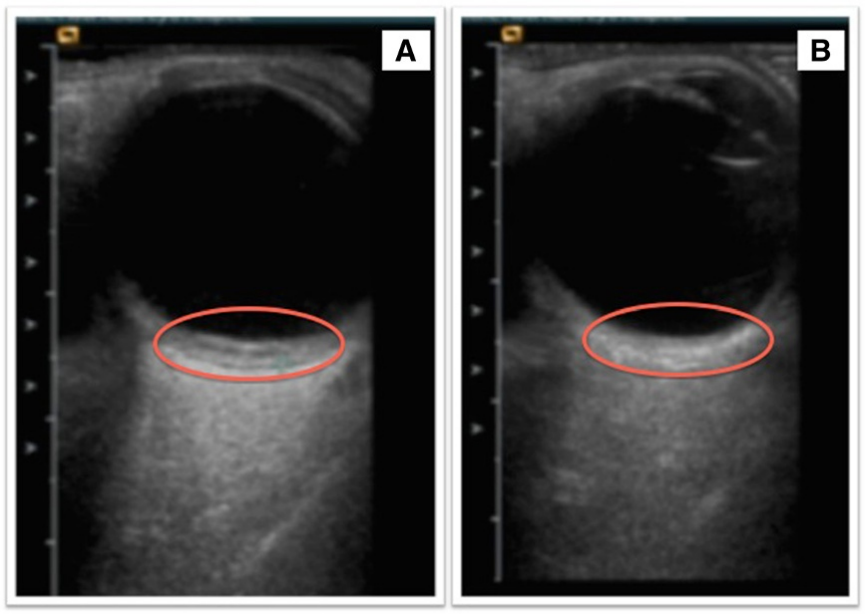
Ultrasound B-scan images of the right eye. Pre-treatment showing presence of nodular sessile swelling in the temporal quadrant (A) and resolution of the sessile swelling after treatment (B).
